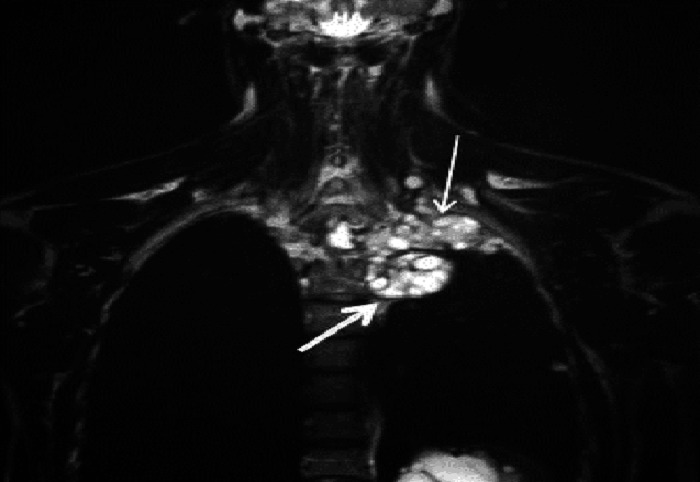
Coronal T2 weighted MRI showing hydatid cysts of the pulmonary apex (thin arrow) delineated at the inferior border by an hypointense rim (thick arrow)
